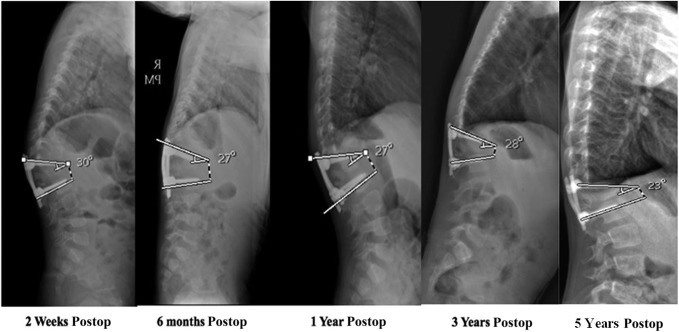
Lateral plain radiograph imaging demonstrating sustained kyphotic deformity correction through 5 years postoperatively. (Reproduced with permission from the Children's Orthopaedic Center, Los Angeles.)
